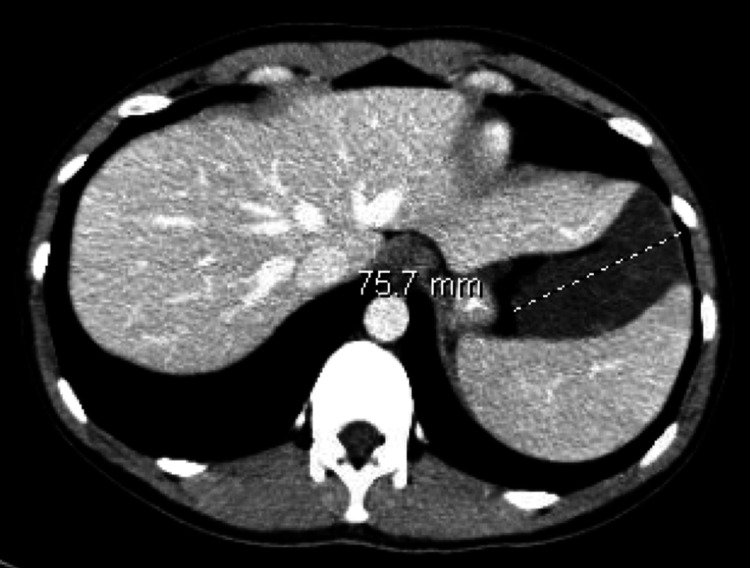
CT Abdomen/Pelvis With Intravenous (IV) and Oral Contrast Left-sided subdiaphragmatic cystic lesion measuring 7.6 cm in transverse dimension is noted below the diaphragm between the liver and the spleen. CT, Computed tomography
